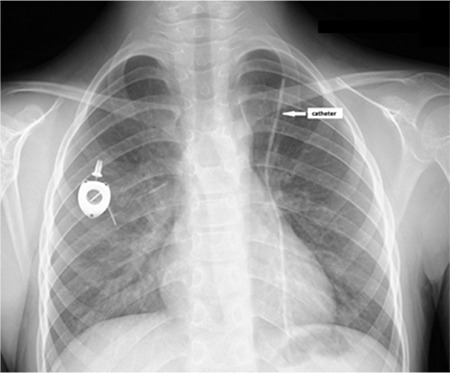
Chest X-ray showing disconnection of the catheter from its reservoir.
